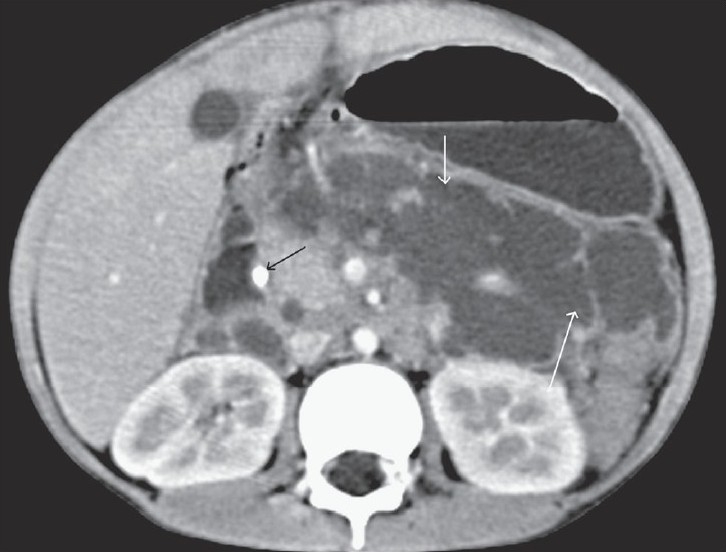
Transaxial contrast-enhanced CT scan shows a large, loculated retroperitoneal collection displacing the stomach anterosuperiorly (white arrows) with a pseudowall and septae and a calculus in the distal part of CBD (black arrow)
